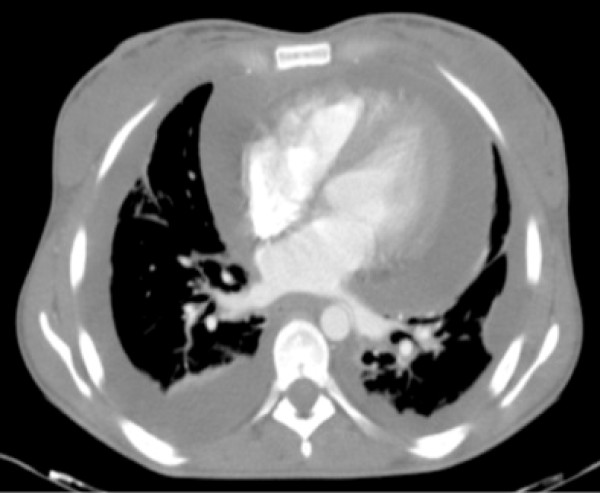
CT scan showing pleura-pericardial effusion.
